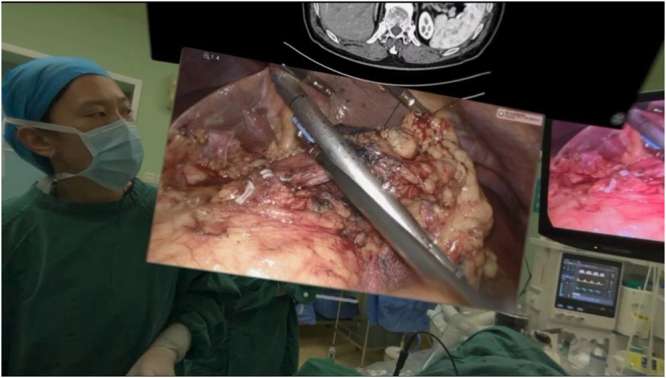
In patients with hypertrophic mesangial tissue, CT examination images during the operation are more conducive to tumor location.
